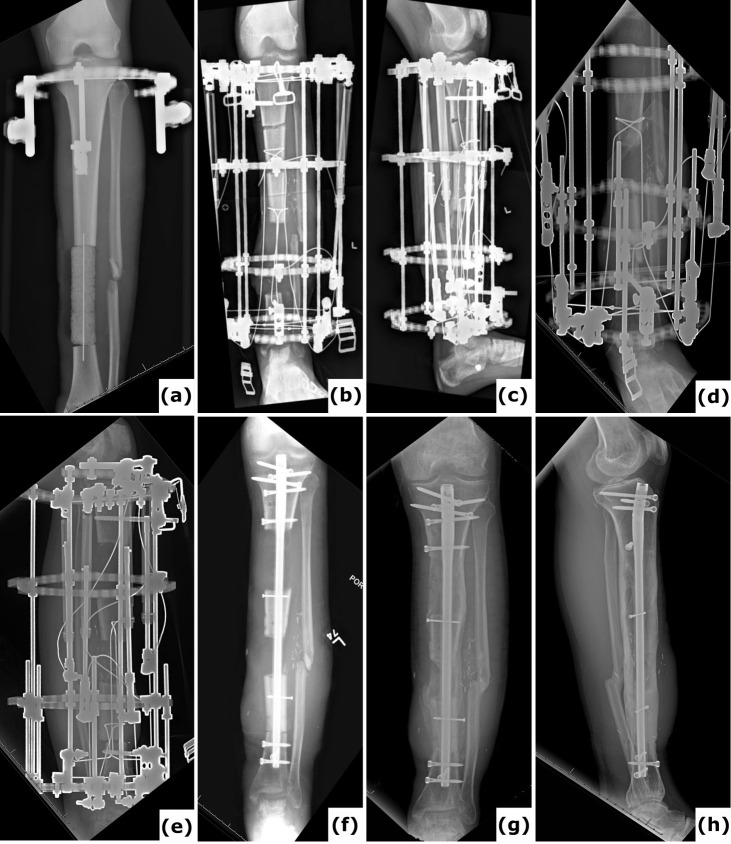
Trifocal balanced cable-transport and then nailing. (a) Anteroposterior radiograph of a 28-year-old patient with a Gustilo-Anderson type III open tibia and fibula fracture including 12.0 cm bone loss and 1.5 cm shortening from a motorcycle crash. During a first surgery, a temporary PMMA spacer was placed in the defect site and the bone ends were prepared for bone transport. (b–c) Anteroposterior and lateral radiographs after a tandem trifocal balanced cable transport external fixator were applied. Note there are two sets of cables and two distinct osteotomy sites for transport. (d–e) Anteroposterior radiograph with transport complete and additional lengthening at the proximal osteotomy site in process. (f) Anteroposterior radiograph after conversion to intramedullary fixation. The conversion was done shortly after the transport, and lengthening was completed. (g) Anteroposterior radiograph 7 months after the start of reconstruction, showing rapid progression of healing. (h) Lateral radiograph 2 years after the start of reconstruction, showing solid and mature healing at all sites.
